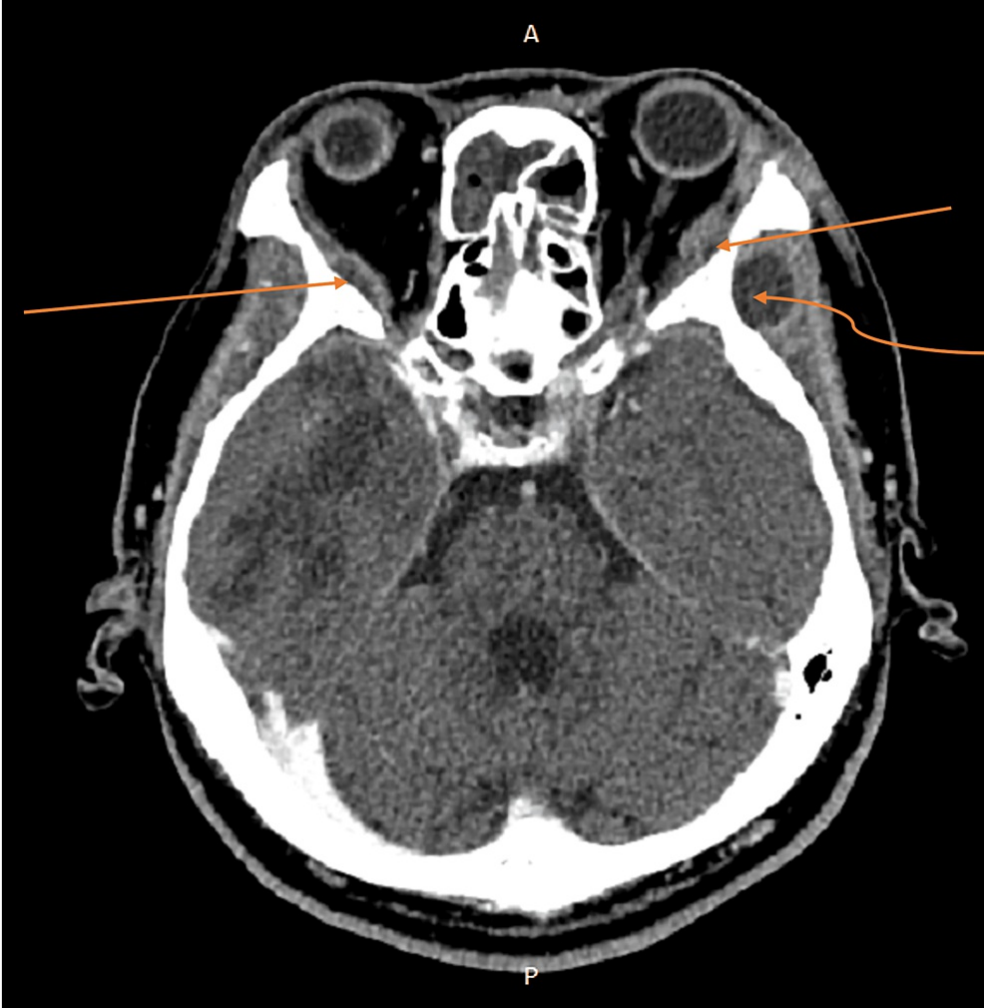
Repeat CECT six weeks after systemic antibiotic therapy shows the development of focal rim enhancing collections at the left lateral extraconal space measuring 0.6 cm x 1.6 cm and at the right lateral extraconal space measuring 0.5 cm x 1.6 cm (straight arrow). Also seen is the development of focal rim enhancing collection at the left temporalis measuring 1.6 cm x 1.3 cm (curved arrow). CECT: Contrast-enhanced computed tomography
